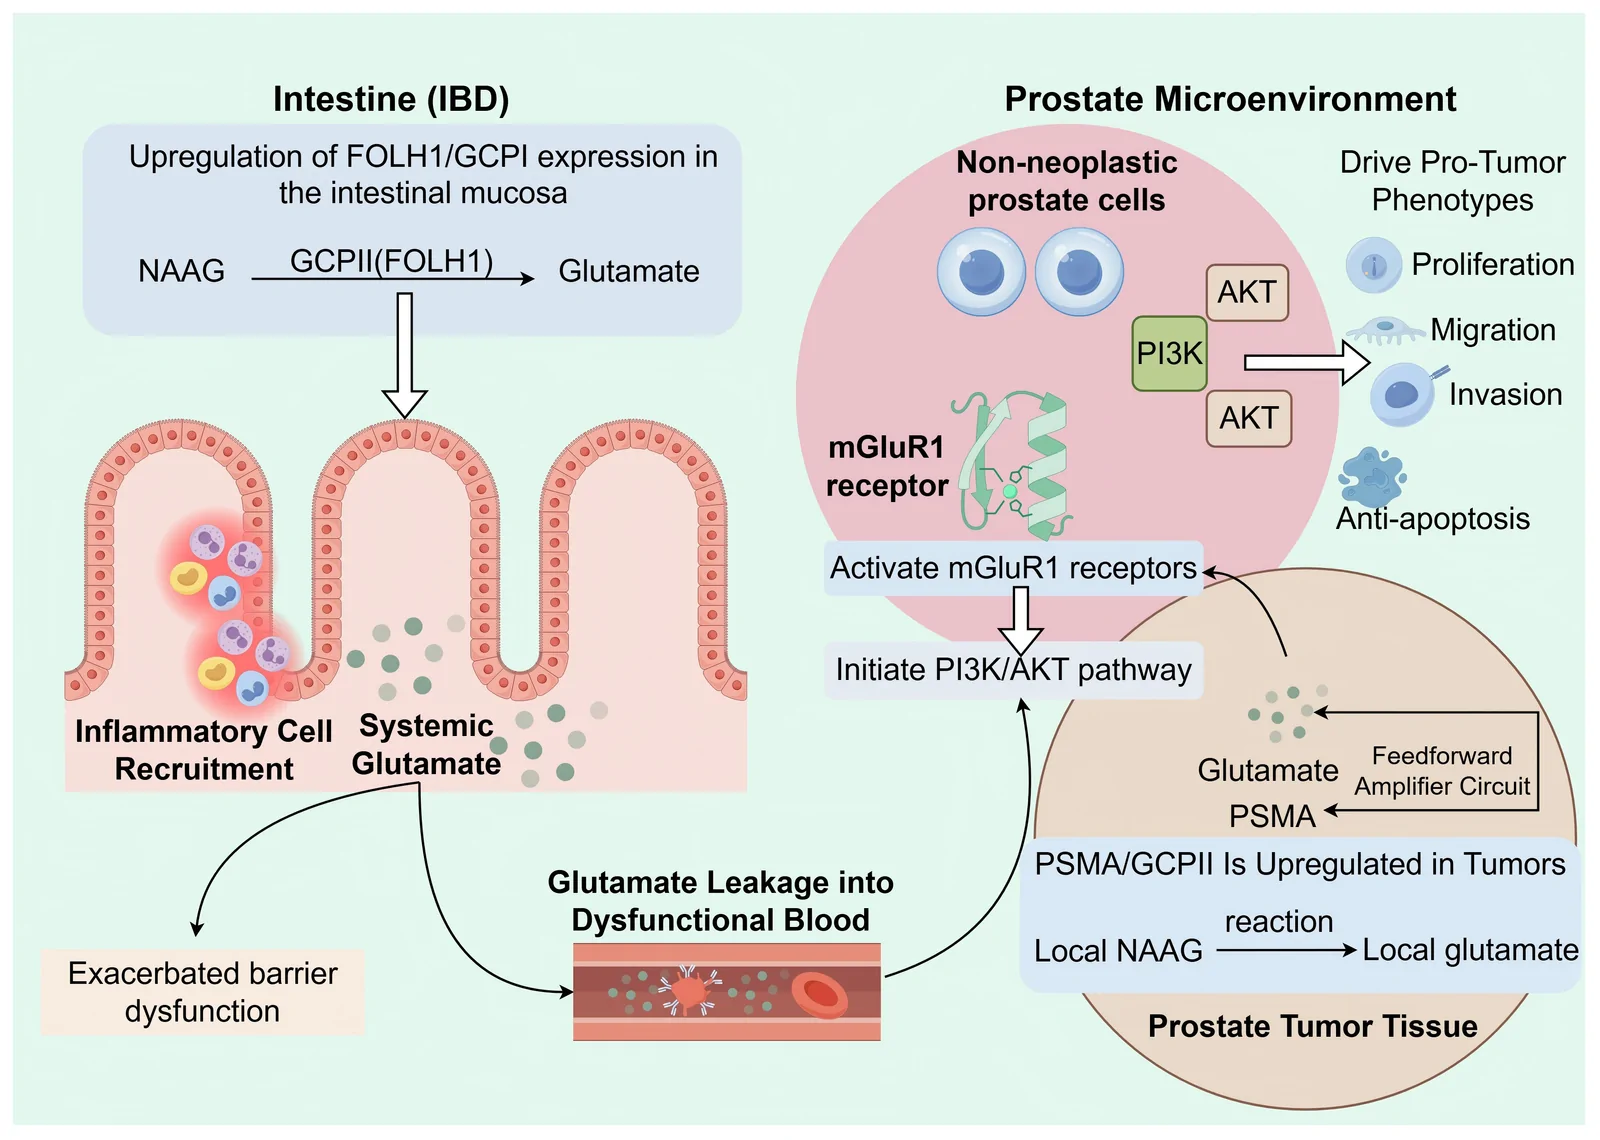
The GCPII–Glutamate–mGluR1 axis as a shared molecular bridge linking intestinal FOLH1 upregulation to prostatic PSMA-Driven carcinogenesis. Glutamate carboxypeptidase II (GCPII), encoded by the FOLH1 gene, is a type II transmembrane metallopeptidase known by three tissue-specific names: PSMA in the prostate, FOLH1 in the intestine, and NAALADase in the brain—all products of the same gene. In IBD, FOLH1 is upregulated in inflamed mucosa and functionally drives intestinal inflammation. In prostate cancer, PSMA is overexpressed on malignant epithelial cells, where its cleavage of N-acetylaspartylglutamate (NAAG) releases glutamate, which activates mGluR1 and PI3K/AKT signaling to promote proliferation and invasion. Serum glutamate correlates positively with Gleason score. Whether intestinally derived glutamate contributes to this circulating pool and primes the prostate for transformation remains a testable hypothesis. A critical knowledge gap is whether the same IBD patient who later develops PCa exhibits both intestinal FOLH1 and prostatic PSMA overexpression in a temporally coordinated manner. PSMA-PET imaging offers a potential approach to test this hypothesis. FOLH1, folate hydrolase 1; GCPII, glutamate carboxypeptidase II; IBD, inflammatory bowel disease; mGluR1, metabotropic glutamate receptor 1; NAAG, N-acetylaspartylglutamate; NAALADase, N-acetylated-alpha-linked acidic dipeptidase; PCa, prostate cancer; PET, positron emission tomography; PI3K, phosphoinositide 3-kinase; PSMA, prostate-specific membrane antigen.
